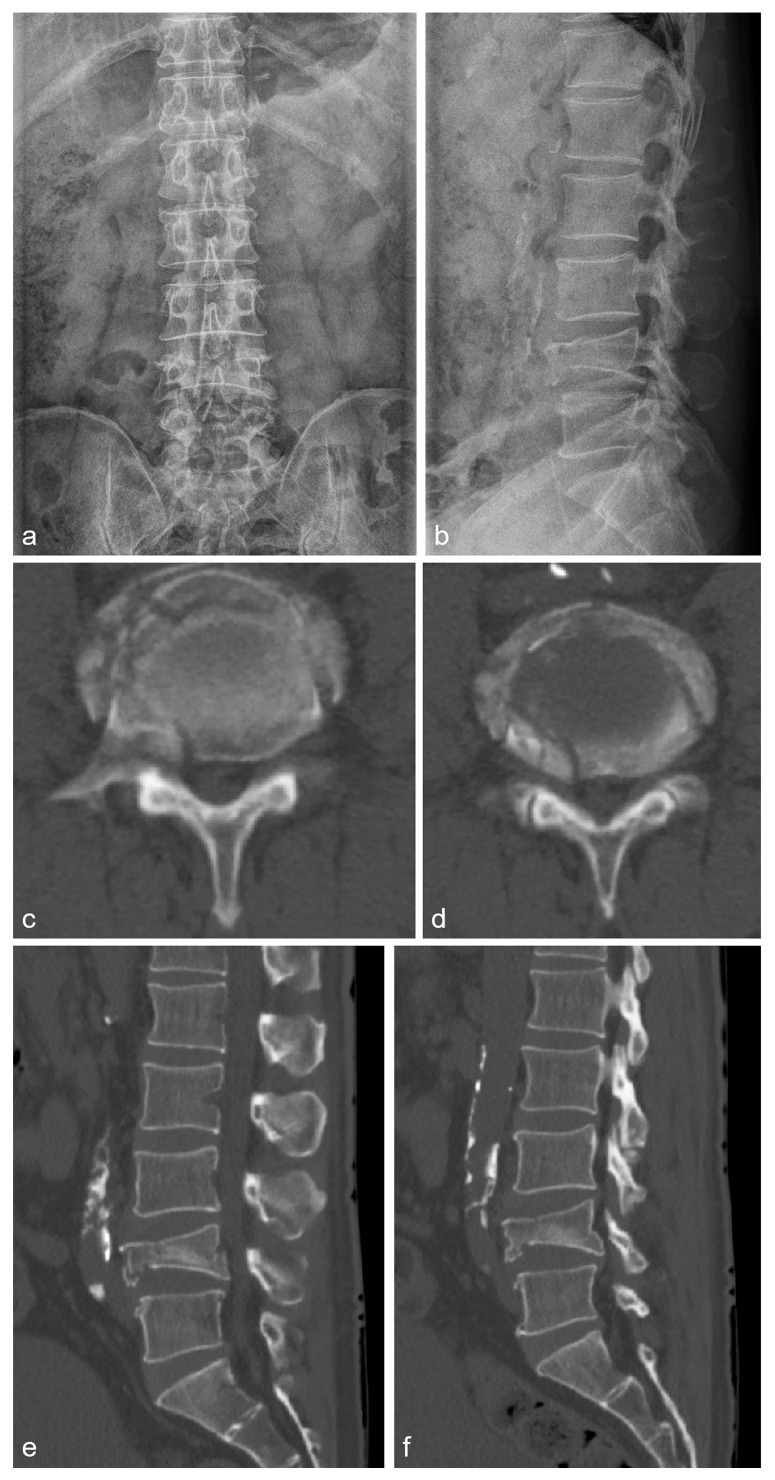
(a,b) Radiographic image before the first surgery showing burst fracture at L4. (c–f) CT image before the first surgery showing central canal stenosis at the L4/L5 level.
